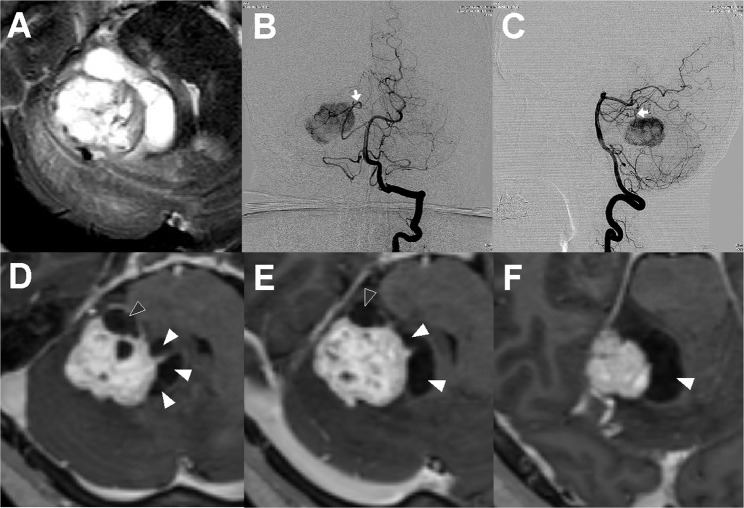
A case of a hemangioblastoma with Type Cm. (A) Brain T2-weighted magnetic resonance imaging (MRI) shows multiple cystic components in right cerebellum and middle cerebellar peduncle. Profound peritumoral edema was noted with brain stem compression. (B,C) Cerebral angiography revealed tumor feeders come from a superior cerebellar artery located superomedial to the tumor mass. (D–F) T1-weighted MRI shows about 3 cm sized strong-enhanced mass with both solid and cystic components. Multiple cystic components were located medial to the main solid mass. Some of the cystic wall showed fairly strong enhancement (black arrow heads) whereas majority of the cysts did not (white arrow heads).
